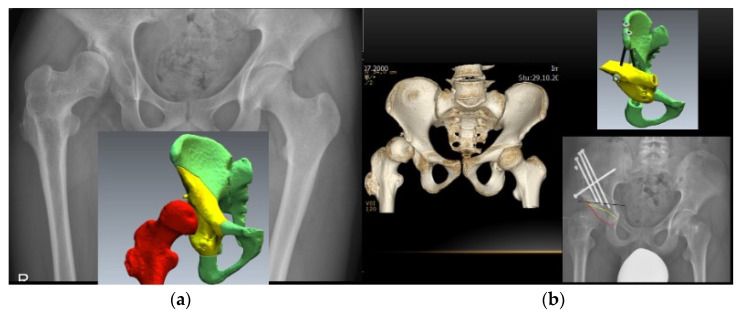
Periacetabular osteotomy (PAO). (a) preoperative X-ray and patient-specific 3D preoperative planning; (b) preoperative 3D CT, planned position of the 3D reoriented acetabular fragment and postoperative X-ray after PAO according to the preoperative patient-specific 3D plan.
